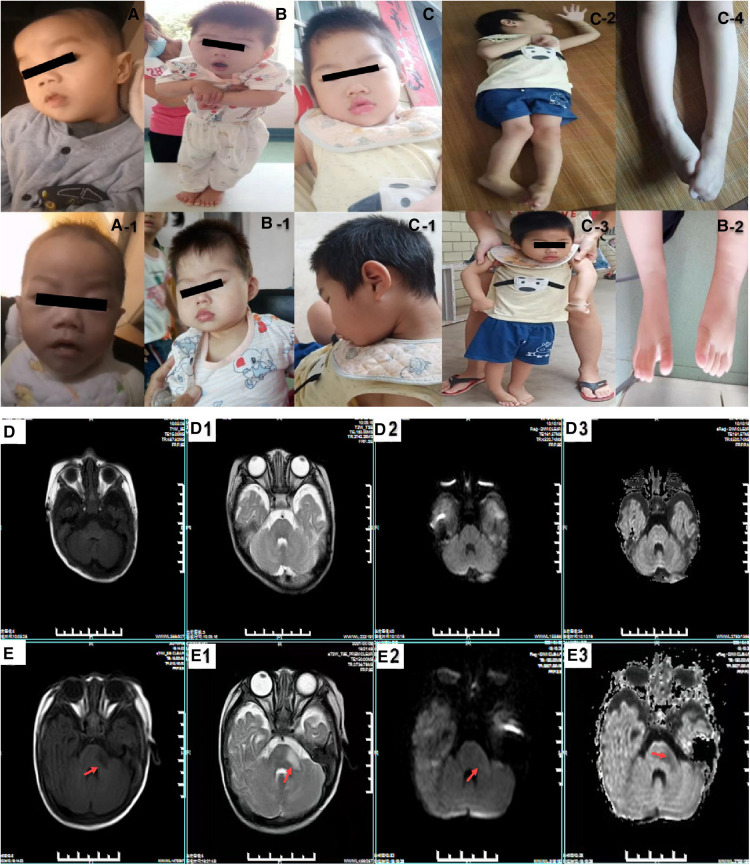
The clinical features of the probands with Allan-Herndon-Dudley Syndrome. (A,A-1) show the phenotypic characteristics of patient 1: fixed facial expression and neck muscle weakness. (B–B-2) show the phenotypic characteristics of patient 2: fixed facial expression and muscle weakness. (C–C-4) show the facial features and muscle weakness and special posture of patient 2's elder brother. (D–D3) show the cranial MRIs of patient 1 (normal). (E–E3) are patient 2 cranial MRIs. (E,E–E3) represent T1WI, T2WI, a high b-value DWI and ADC images, respectively. The results reveal a patchy long T1 and a long T2 signal shadow in the left mid-cerebellar peduncle, with a slightly high signal on high b-value DWI and a slightly low signal on ADC images.
